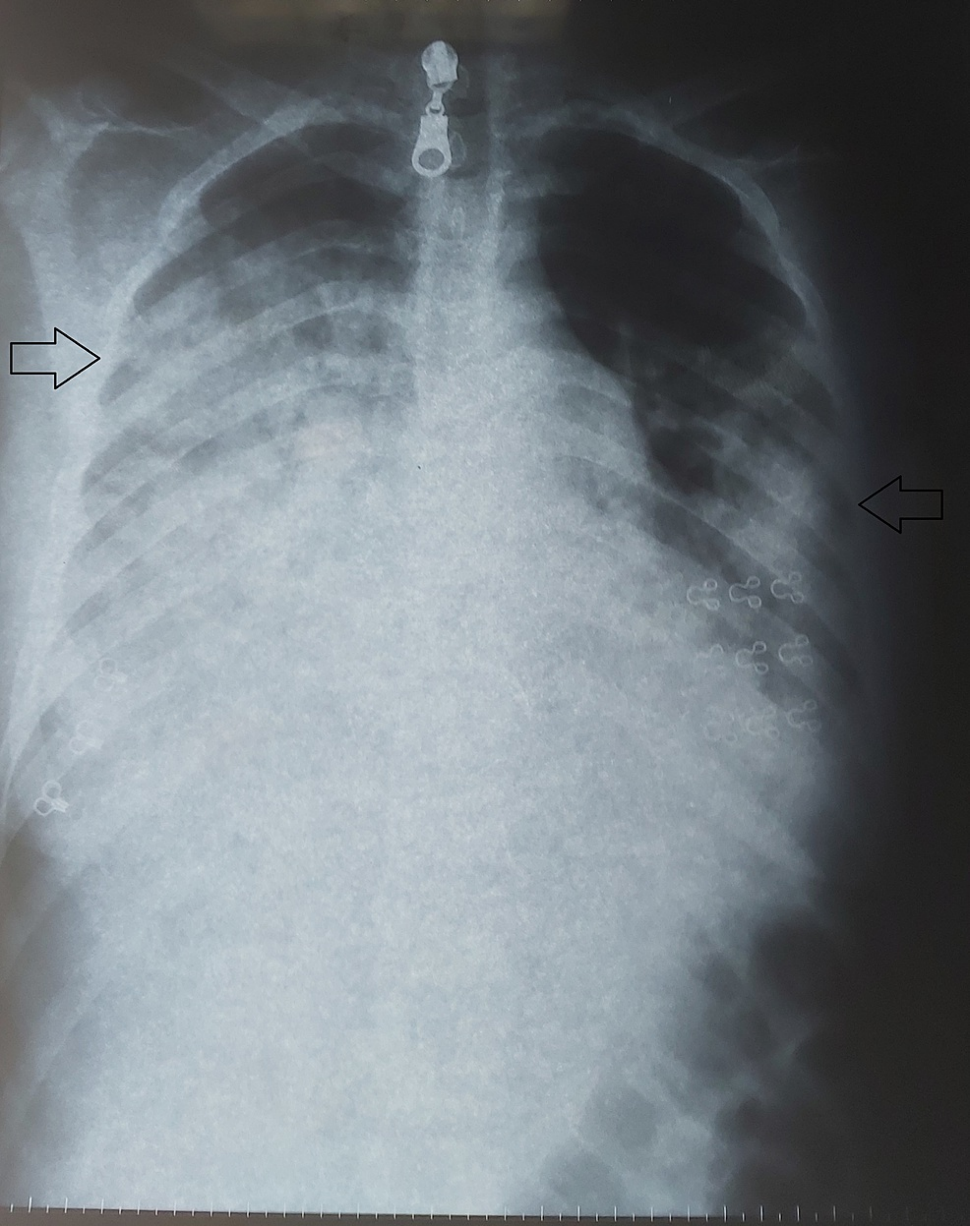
Posteroanterior chest radiograph demonstrates extensive alveolar and interstitial opacities
